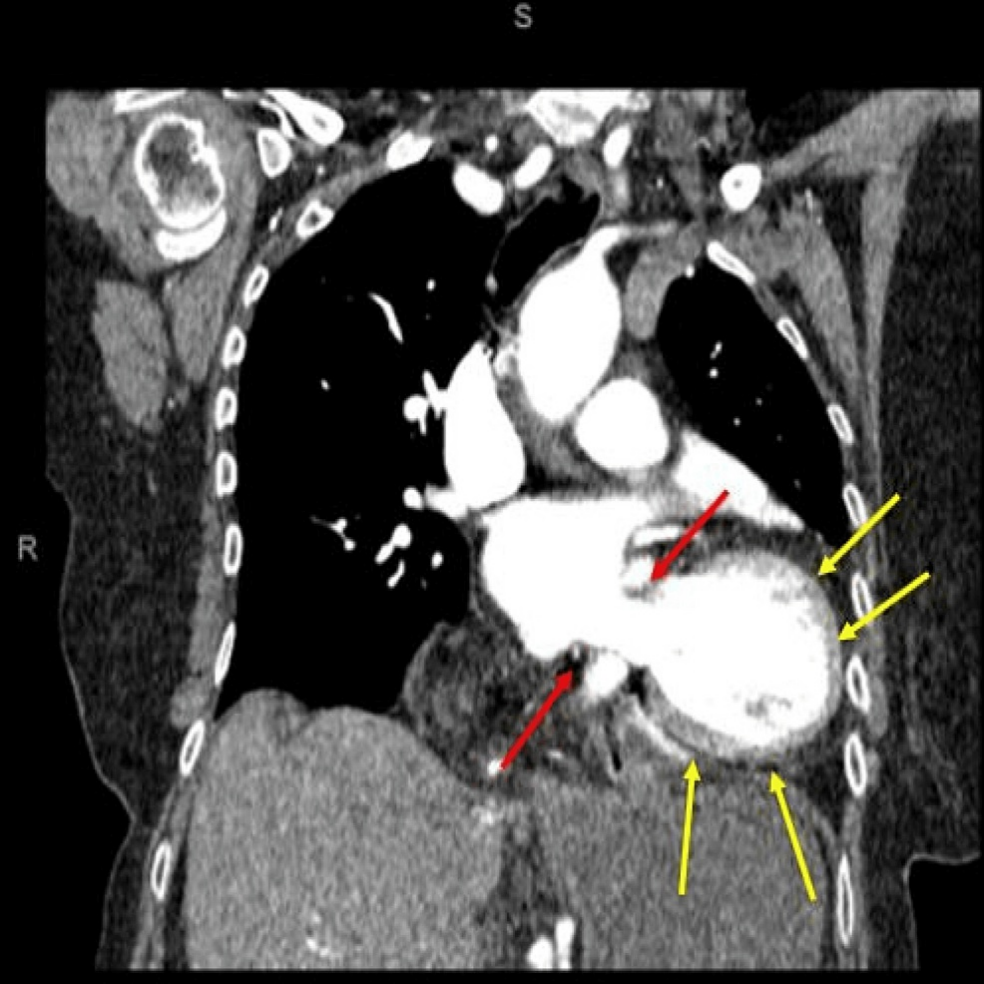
Computed tomogram (CT) chest-abdomen. Notice the gastric volvulus which appears to be associated with rolling paraesophageal type of hiatus hernia (red arrows) and fluid around the gastric component of the intra-abdominal component of the stomach (yellow arrows).
